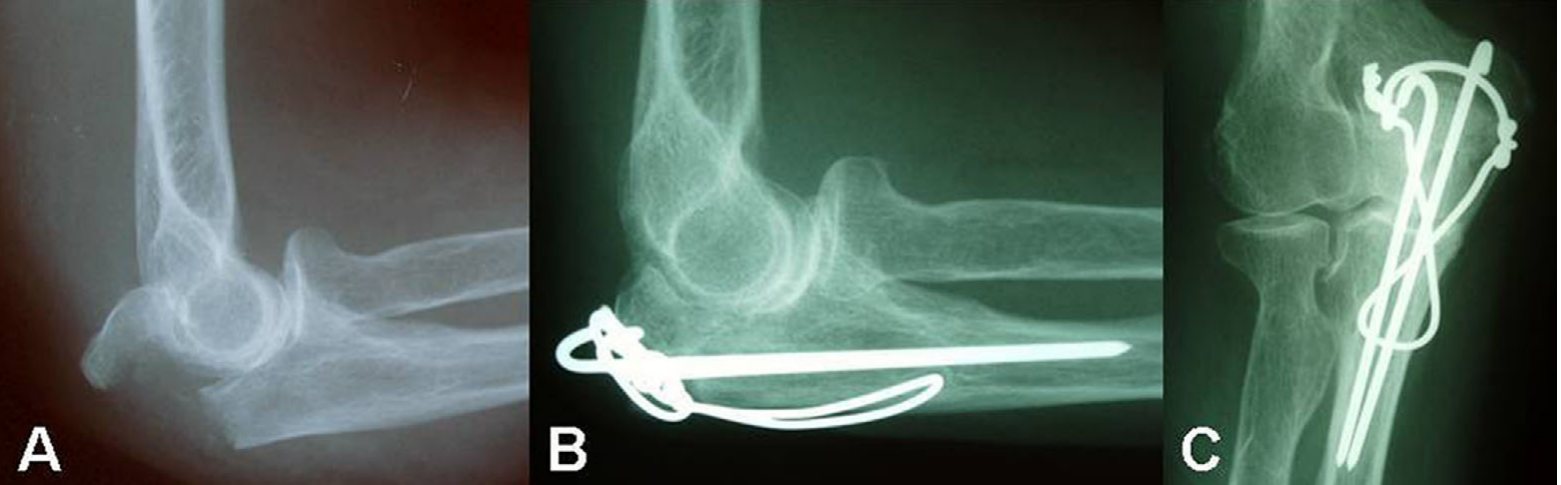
Elbow degeneration and olecranon fracture. Mayo Type IIA fracture of the left olecranon after a fall in a 52-year-old woman (A). Lateral (B) and anteroposterior radiographs (C) at 7 years postoperatively showed signs of subchondral sclerosis and osteophytes formation in radioulnar and ulnohumeral joints.
